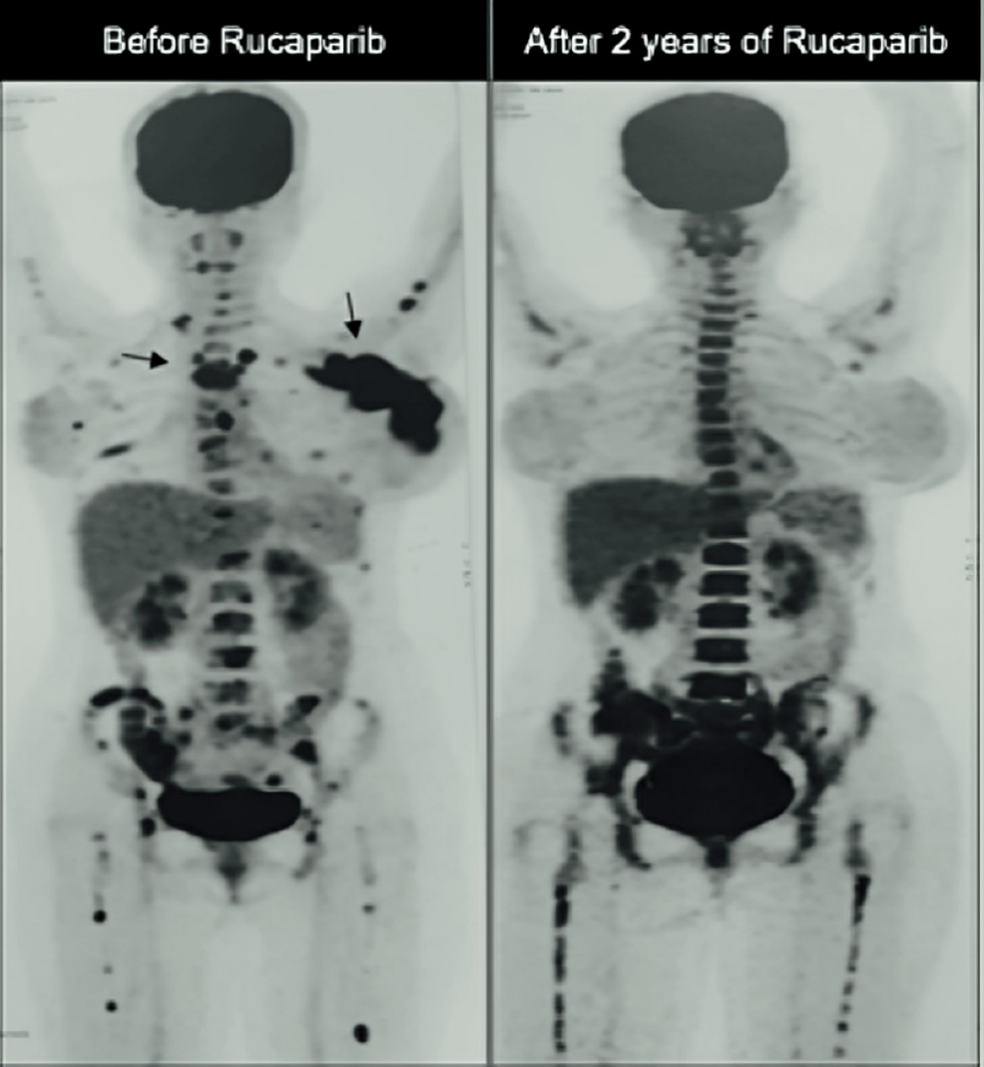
Case 1: Rucaparib Response on PET-CT Scan in BRCA-Mutated MBC Patient BRCA, breast cancer gene; MBC, metastatic breast cancer; PET-CT, positron emission tomography-computed tomography.
